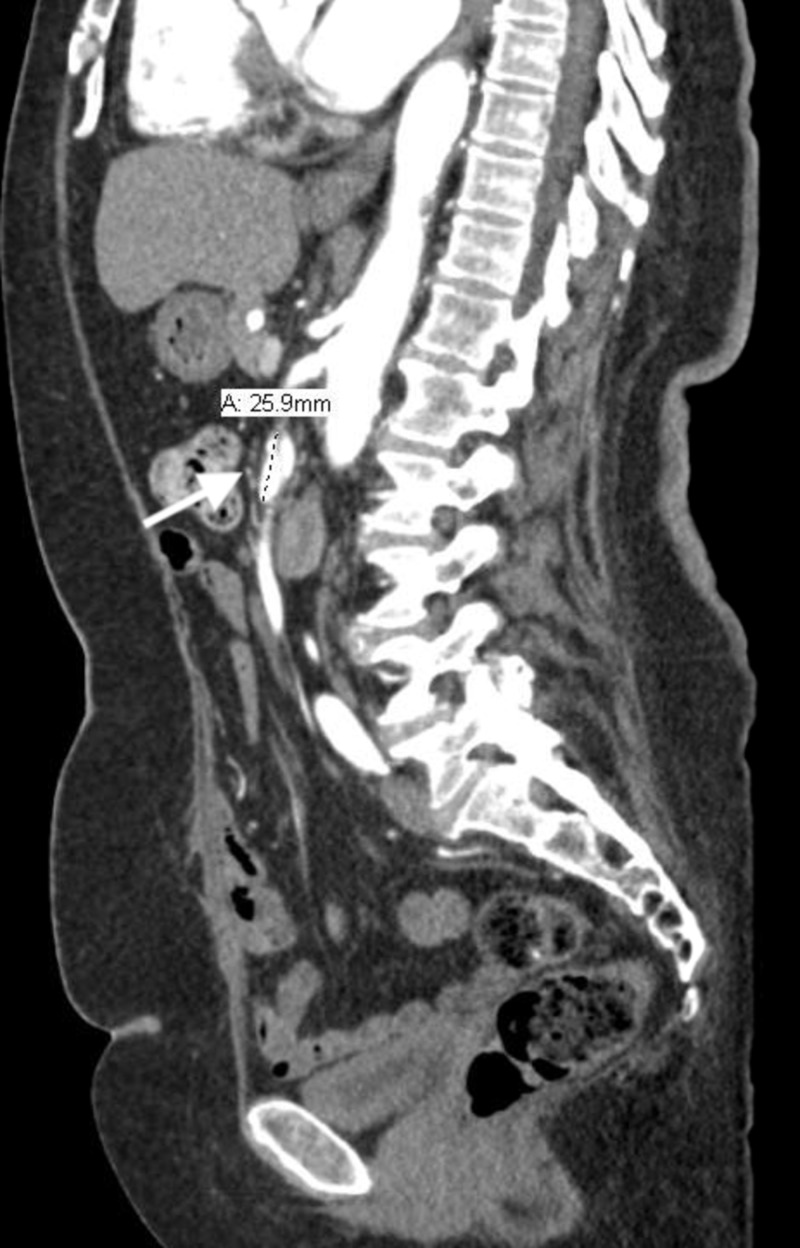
Sagittal view of isolated superior mesenteric artery dissection (indicated by arrow) on a CT angiogram.
